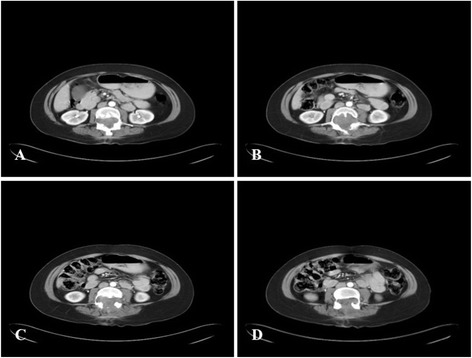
Neuroimaging findings of the GCE from filum terminale. a-d Axial gadolinium-enhanced L3-L5-weighted CT image demonstrated an intradural non-encapsulated heterogeneously enhanced solid mass attached to the filum terminale
